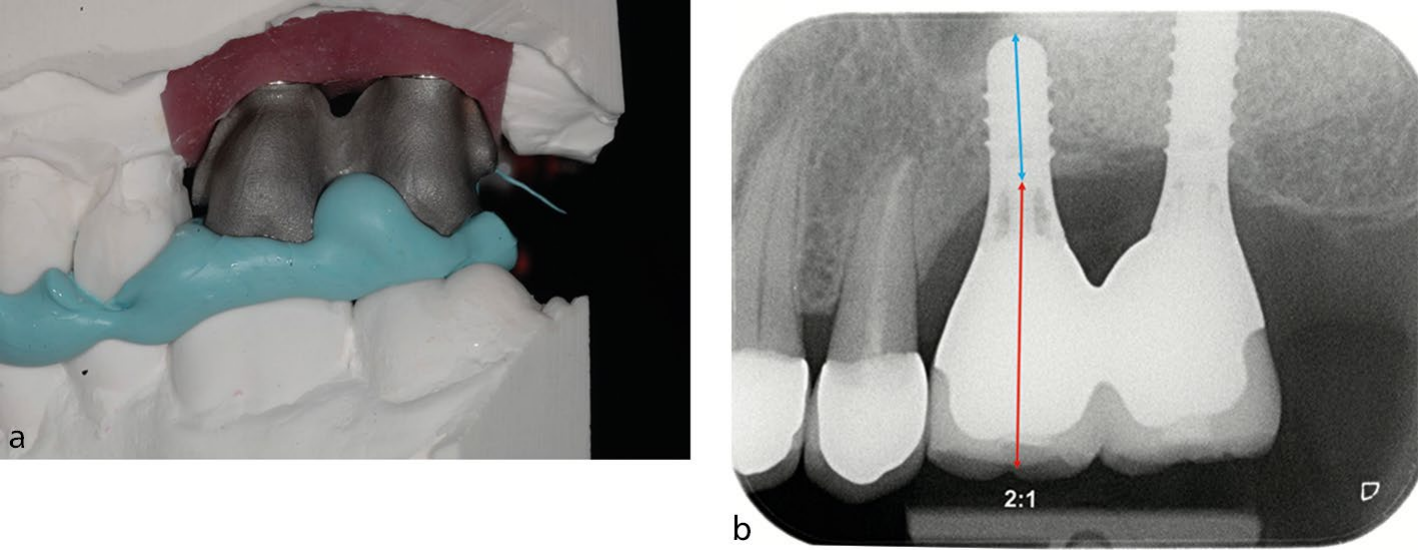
a) Metal substructure for splinted crowns restoring the 26 and 27 implants demonstrating 4-5 mm of vertical resorption (moderate) on dental cast. Minimal horizontal resorption thus straight implant configuration was achieved with the crown in the ideal posterior occlusal relationship without the need for a buccal cantilever. b) IOPA radiograph showing C:I of equal to or greater than 2:1 (26). Augmentation of the left maxillary sinus has enabled longer implant placement; however, increased C:I was still present due to increased IAD
